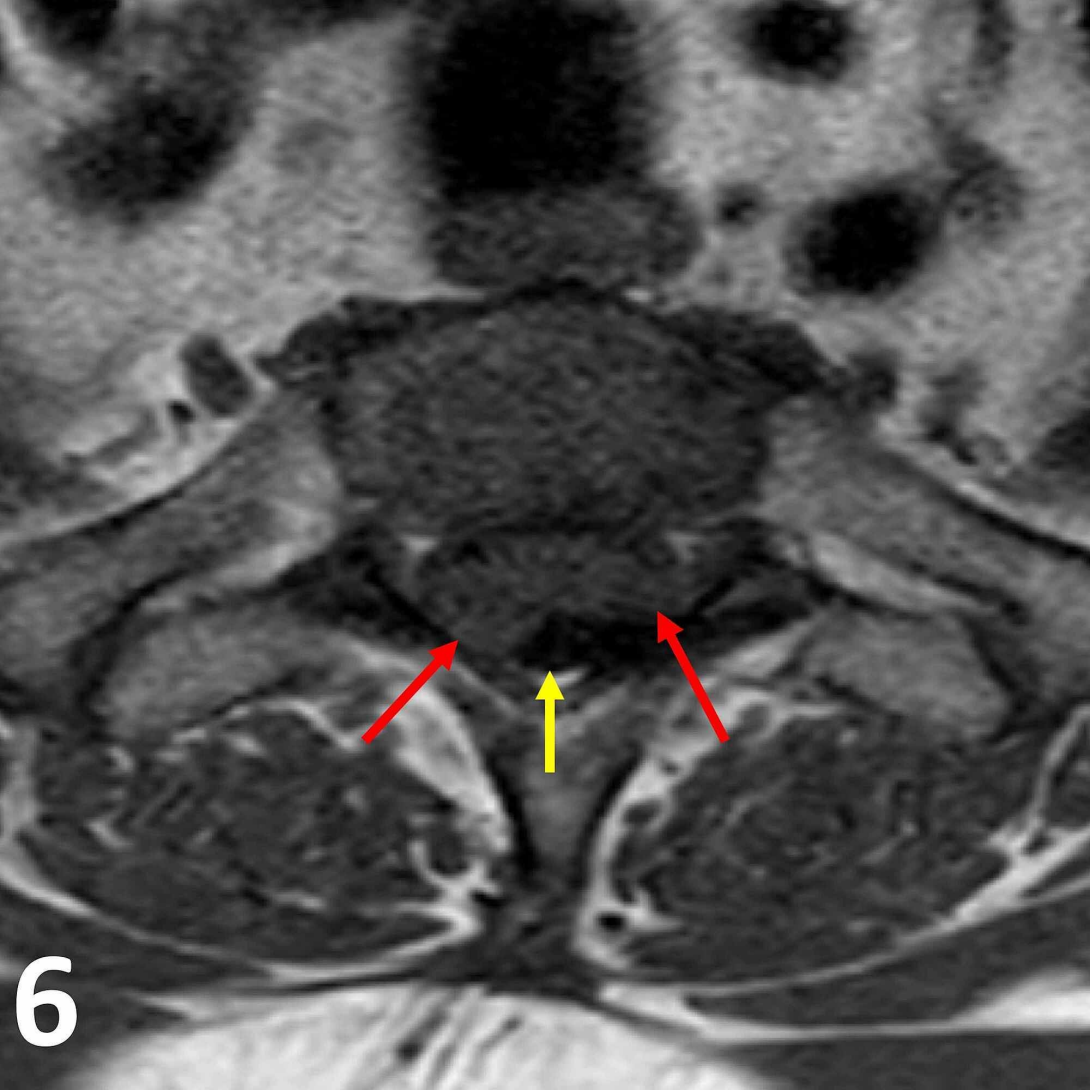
Axial T1 pre-contrast MRI image at the T1/T2 vertebral level. Note the T1 isointense intradural lesions (red arrows) projecting posterolaterally within the thecal sac (yellow arrow).
